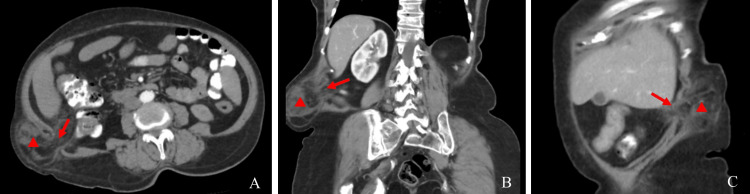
Axial (A), coronal (B), and sagittal (C) enhanced CT acquisitions showing a right inferior lumbar hernia with a neck size of 3.5 cm (arrow) and a hernia sac measuring 8.1cm by 4.9 cm containing adipose tissue and small bowel loops (triangle).
